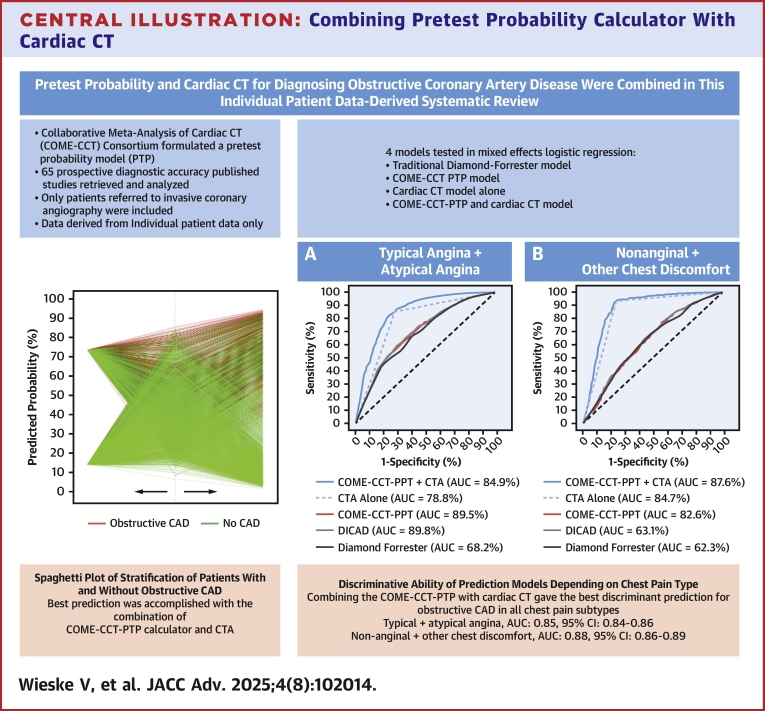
Combining Pretest Probability Calculator With Cardiac CT Abbreviations as in Figure 1, Figure 2.
